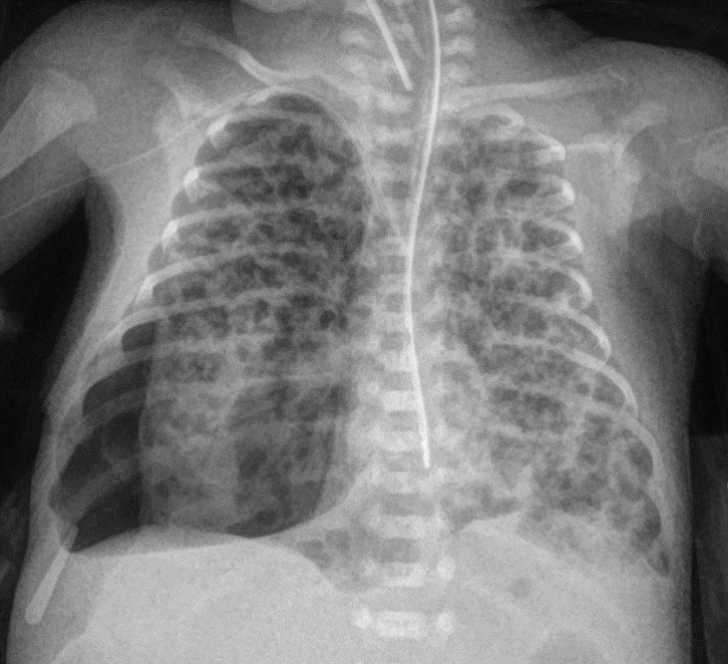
Supine chest radiograph on the 14^th^ day of life shows a lucent right hemithorax, depression of the right diaphragm and leftward mediastinal shift, consistent with a right mild tension pneumothorax.
